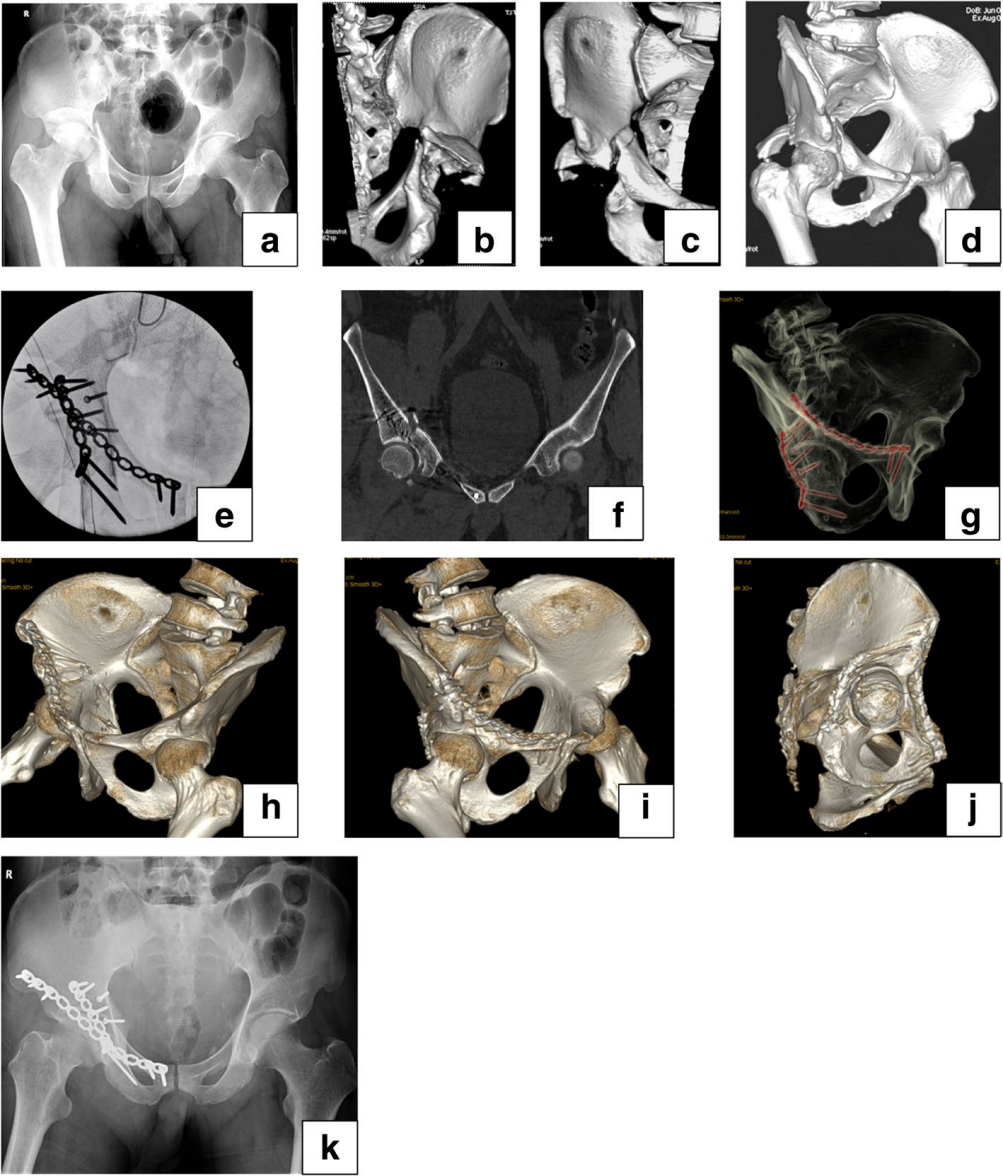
Male, 44-year-old, car accident, a transverse and posterior wall acetabular fracture, treated with posterior plate fixaiton via the K-L approach combined with minimally invasive anterior plate fixation. a Antero-posterior pelvic X-ray shows an acetabular fracture associated with femoral head dislocation. b-d 3D CT images show a transverse and posterior wall acetabular fracture. e Intraoperative views reveal the screws didn’t penetrate into the hip joint. f-j Postoperative 3D CT images reveal satisfactory reduction and fixation of the acetabular fracture. k The postoperative X-ray antero-posterior image at 3 months demonstrate that the fracture healing is achieved
